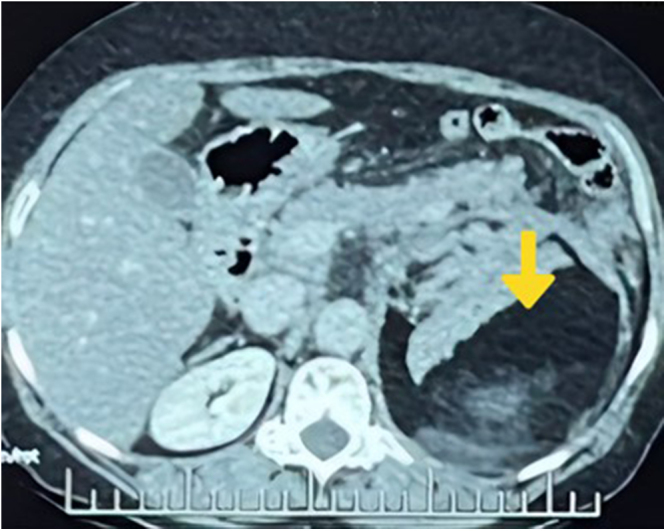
Case 2 – CT scan of the abdomen showing left adrenal myelolipoma (yellow arrow) measuring 11 cm × 10cm × 15 cm.
